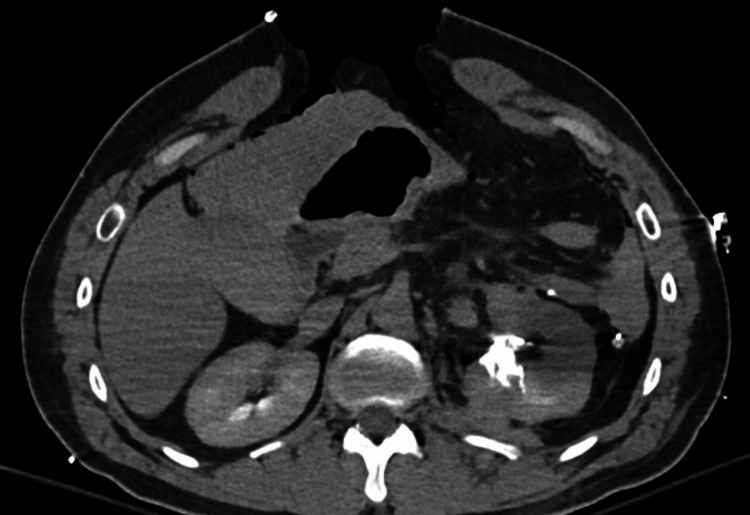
Postoperative CT scan showing a Grade IV left renal injury.
